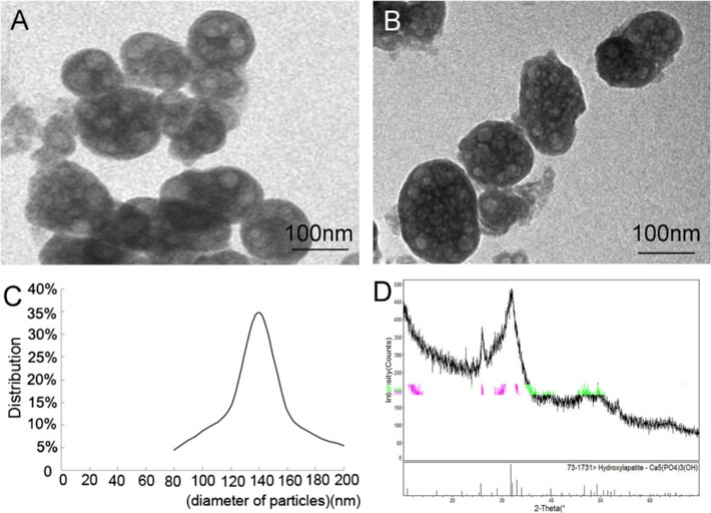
a TEM images of CaP particles. b TEM of CaP particles after being ultrasonicated by ultrasonic cell crushing apparatus. c Particle size distribution of the CaP particles. d XRD patterns of CaP particles
